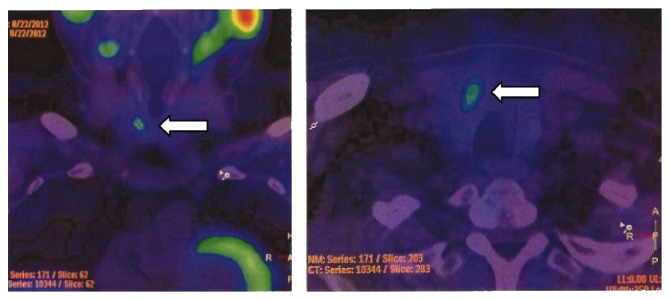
Parathyroid Tc99m-MIB scintigraphy. Images showed an increased uptake of the right superior parathyroid gland on the 150 min delayed image compared with the early 15 min image (white arrow).
